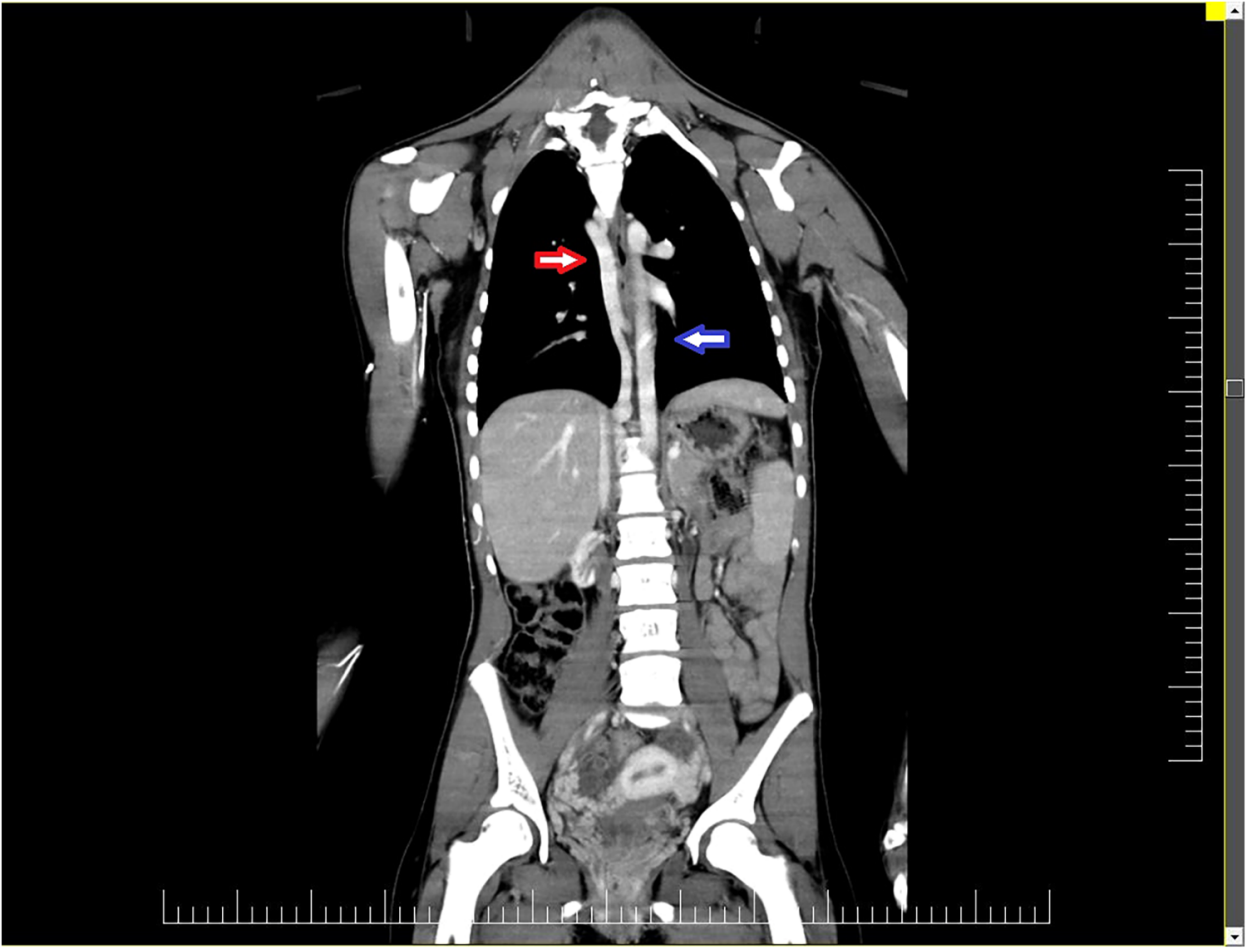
CT angiogram revealed numerous thrombosis sites. The superior vena cava displayed an area of complete distal thrombosis between the right atrium shedding and the azygos vein cross opening in the superior vena cava, on a length of 2.8 cm, with dilated azygos (red arrow) and hemiazygos veins (blue arrow).
